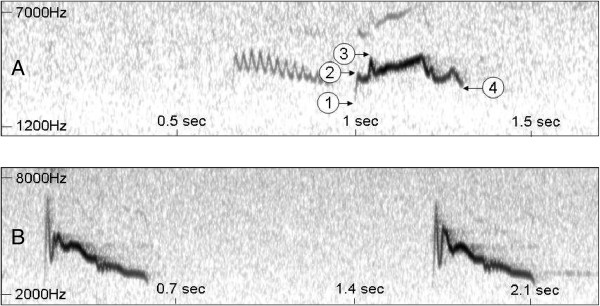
Typical sonograms of the calls of the highland
